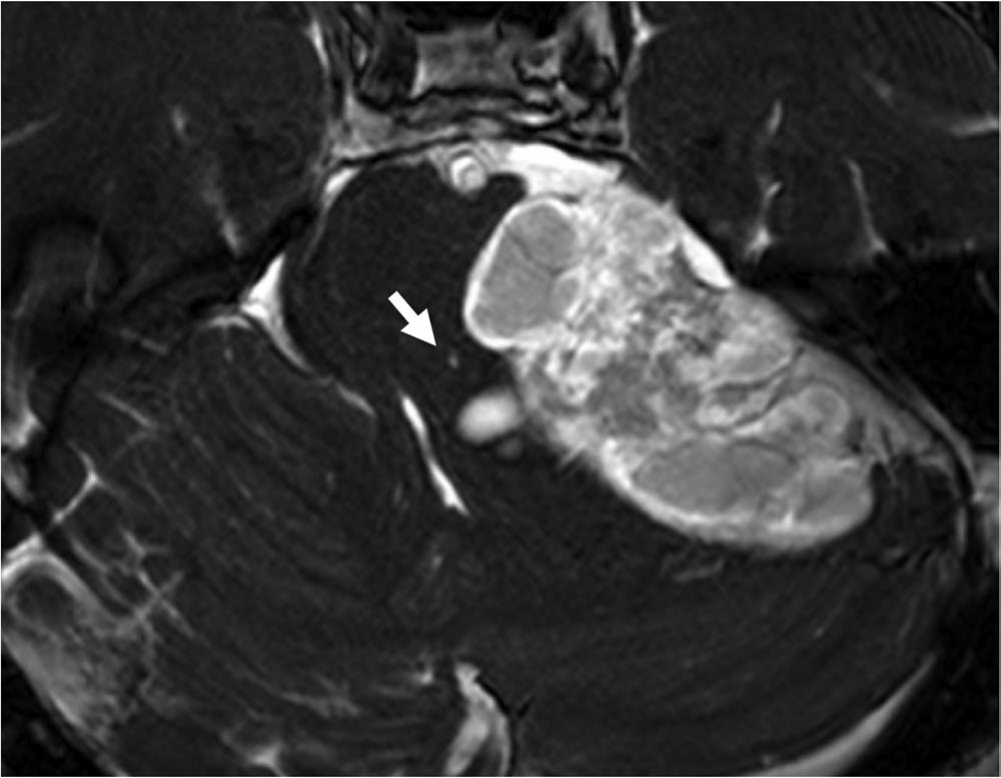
Representive MRI of the focal hyperintensity lesion (FHI) in the brain stem. Preoperative Gd-enhanced b-FFE MR image of a 64-year-old man shows a large cystic vestibular schwannoma at the left cerebellopontine cistern. A FHI can be observed clearly in the dorsal brain stem (arrow).
